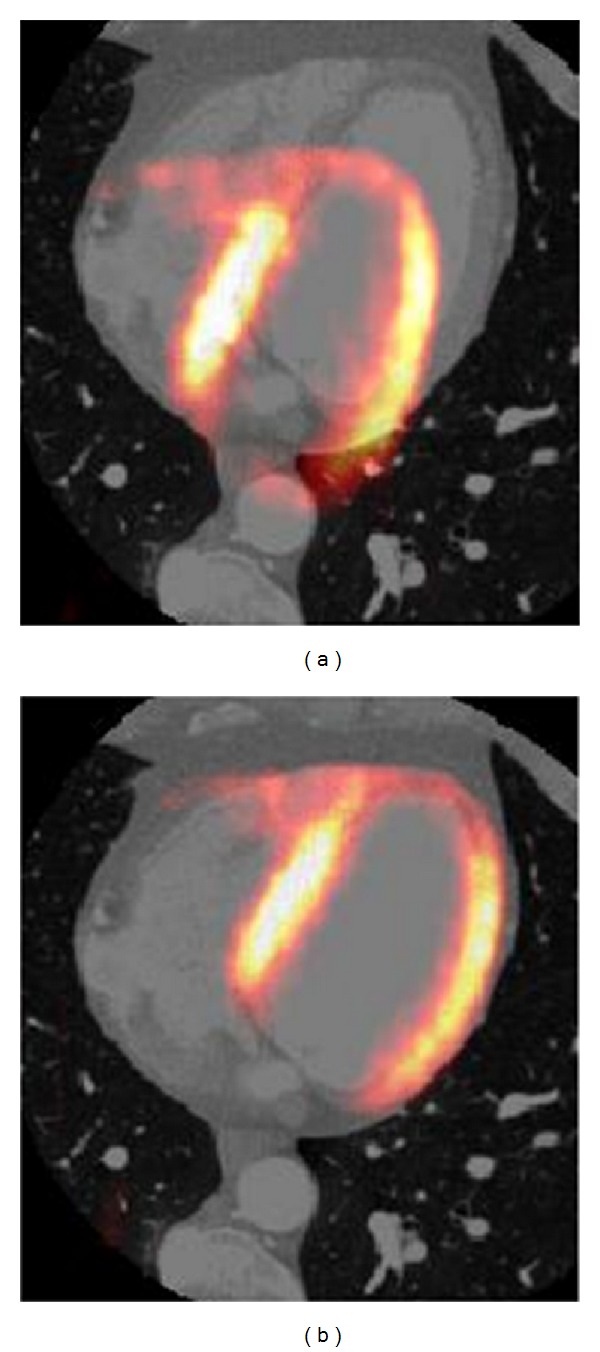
Example of misaligned (a) and aligned (b) transaxial PET and CT images corresponding to dataset 1.
